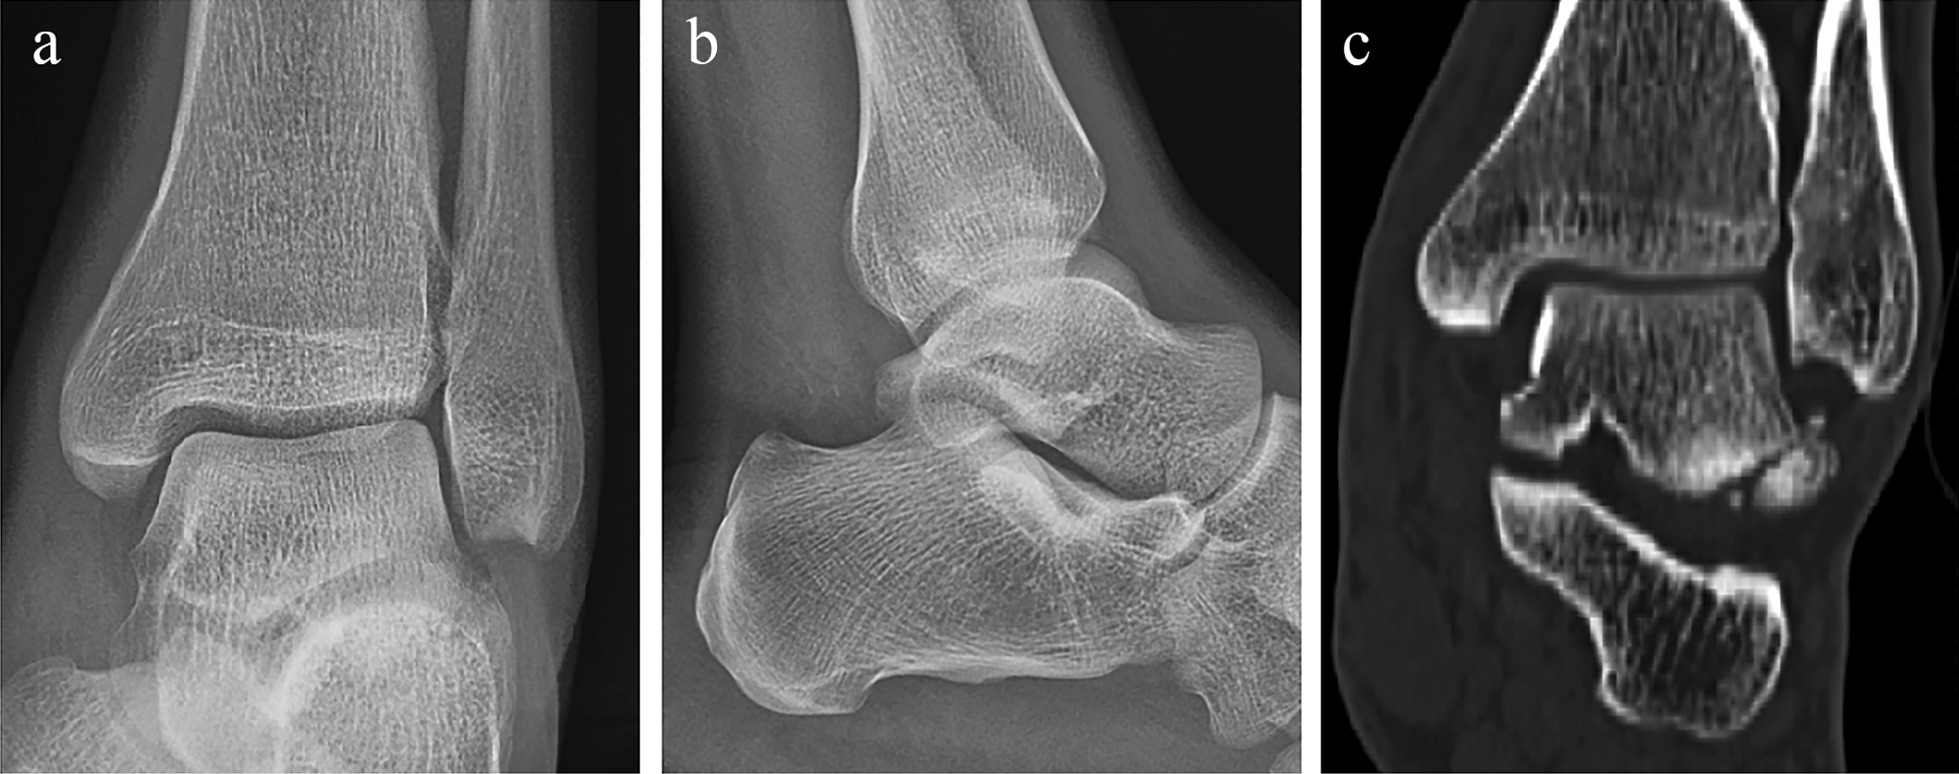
The preoperative imaging data of case 2. a Anteroposterior and b lateral radiograph of the injured ankle showed no obvious fracture of the ankle. c Computed tomography scan revealed fracture of the lateral process of the talus with several chip fragments on both fibular and calcaneal articular surfaces of the talus. On the medial side, soft tissue swelling could be observed around the malleolus medialis
